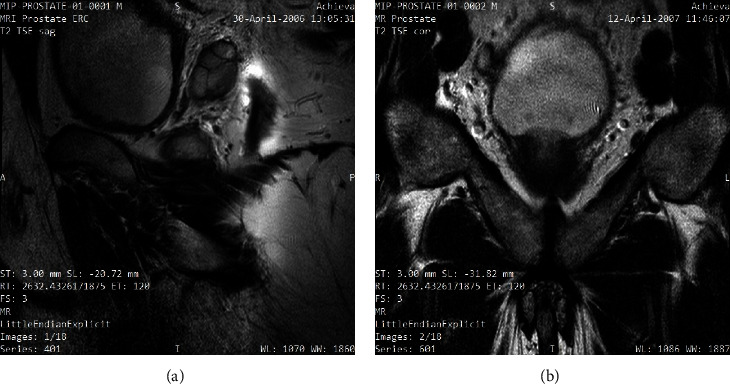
Sample MRI images of: (a) healthy prostate and (b) infected prostate.
